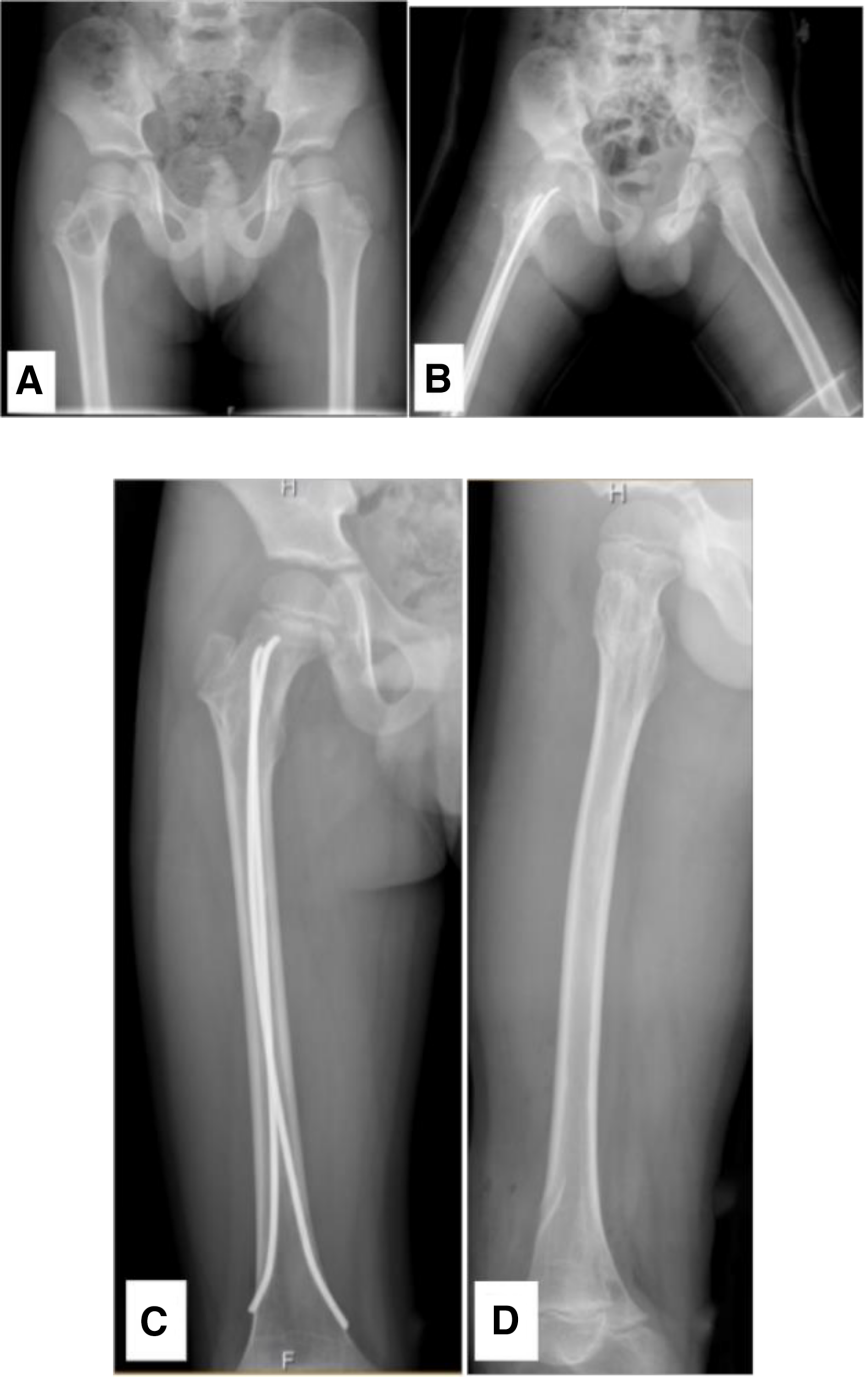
A 6.28-year-old male diagnosed with a SBC in the proximal right femur. (A) Preoperative radiograph. (B) Radiograph taken 3 days post-biopsy, curettage, FIN procedure, and bone grafting. (C, D) Radiographs at 1.4 years post-surgery and following the removal of the internal fixation.
